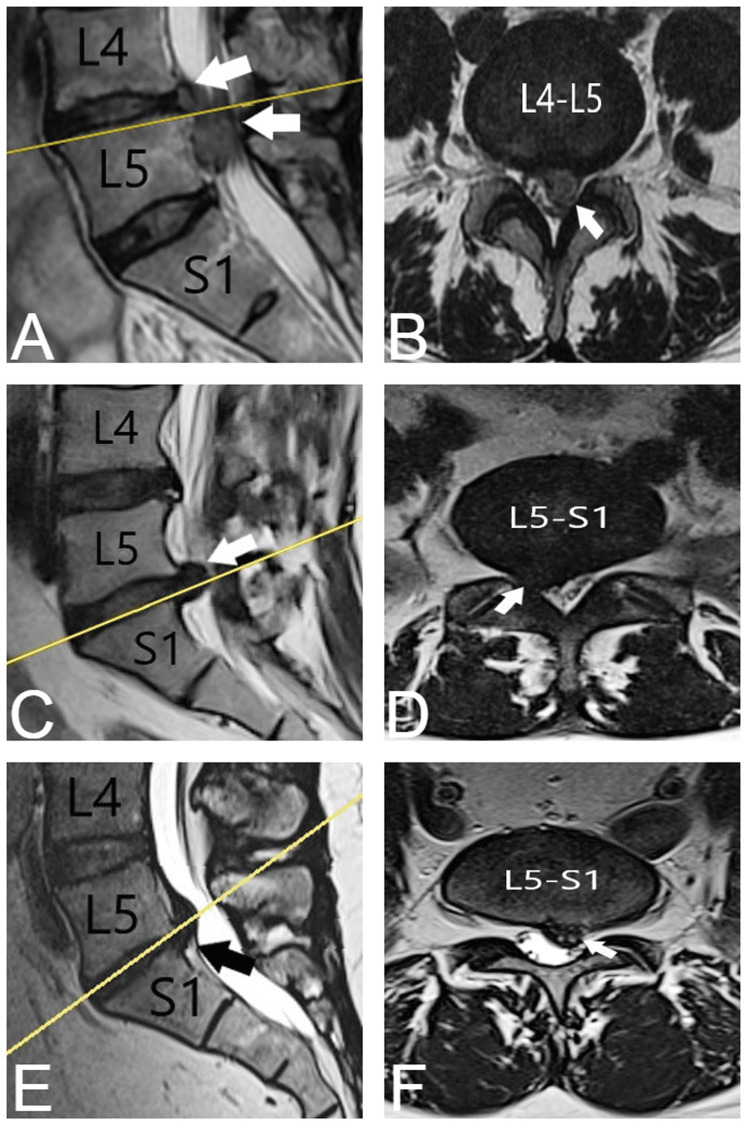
MRI results of patients with LDH (A) Sagittal T2-weighted MRI displaying disc hernia at the level of L4-L5 spinal segment. (B ) Transversal T2-weighted MRI showing a L4-L5 herniated disc. (C) Sagittal T2-weighted MRI displaying L5-S1 disc herniation. (D) Transversal T2-weighted MRI showing a L5-S1 herniated disc. (E) Sagittal T2-weighted MRI displaying L5-S1 disc herniation. (F) Transversal T2-weighted MRI showing a herniated disc at the L5-S1 spinal segment. Arrows displaying the disc herniation.
